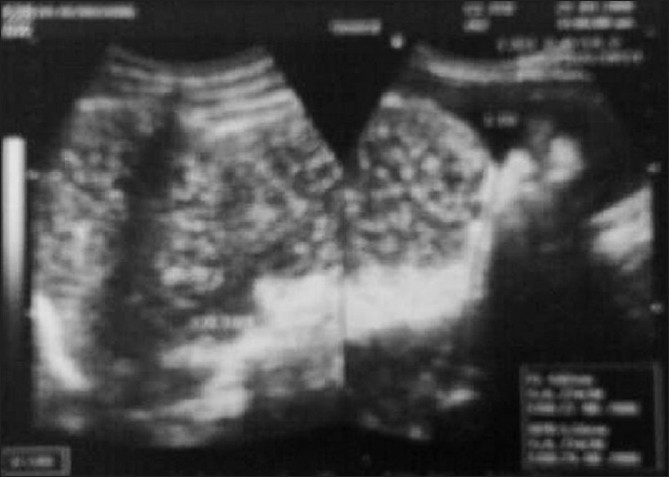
Ultrasonography of our patient, showing peritoneal fluid
